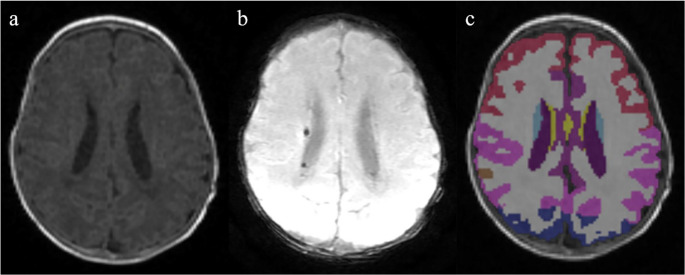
Brain magnetic resonance imaging of a female neonate born at 24 weeks of gestation with images acquired at 40 weeks’ postmenstrual age. (a) Three-dimensional T1-weighted image; (b) susceptibility-weighted image; and (c) segmentation image. Low-grade germinal matrix-intraventricular hemorrhage was observed on brain ultrasonography and susceptibility-weighted imaging. Volumetric analysis showed a volume reduction of −1.93 standard deviations. The Bayley-III test at 48 months confirmed neurodevelopmental impairment, with scores of 65, 67, and 67 in the cognitive, language, and motor domains, respectively. In the segmentation image, red, pink, brown, blue, purple, yellow, violet, sky blue, and gray correspond to the frontal lobe, parietal lobe, temporal lobe, occipital lobe, cingulate cortex, insula, lateral ventricle, caudate, and white matter, respectively.
